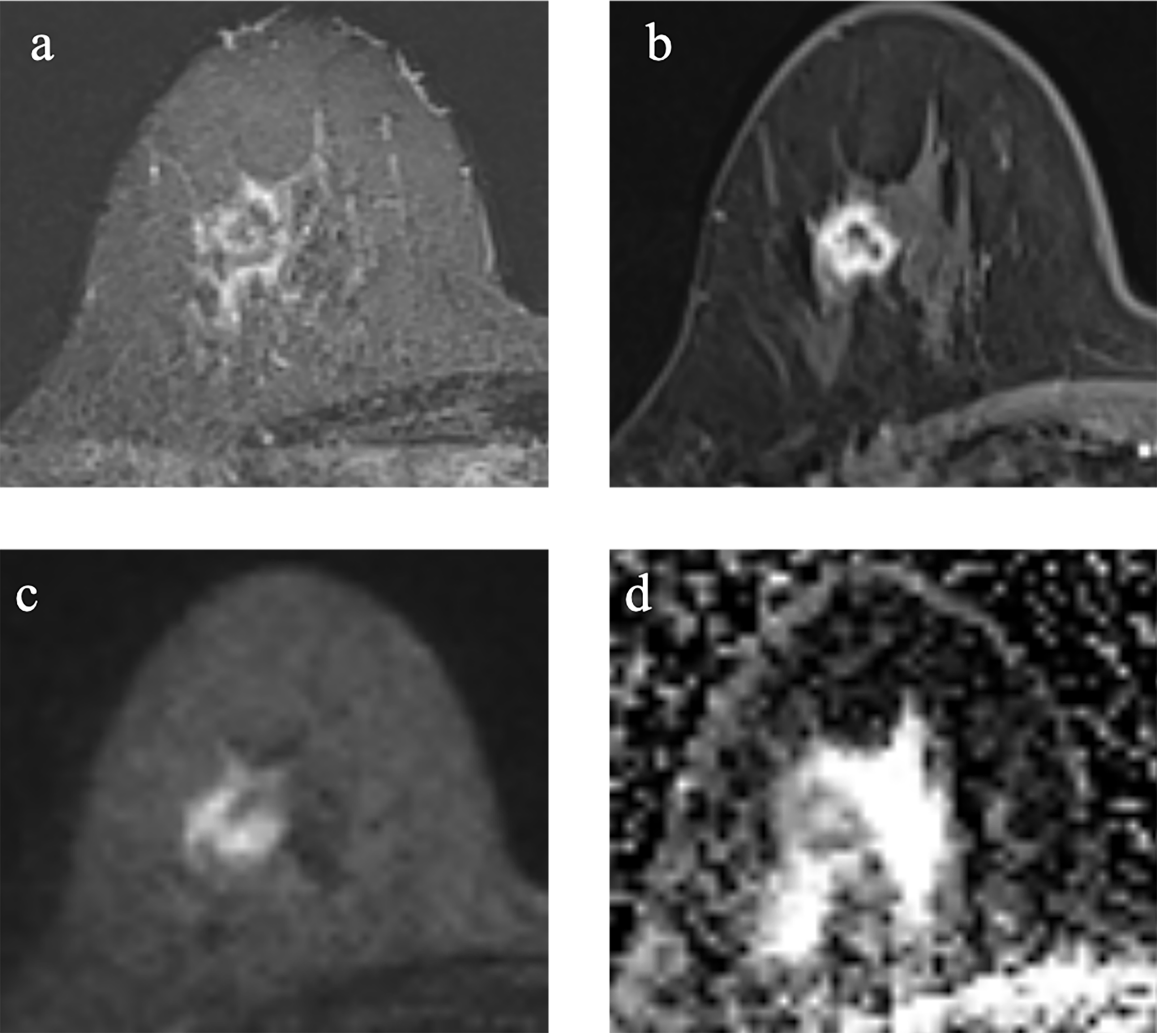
Example images of a 70-year-old woman with TNBC without TP53 mutation in the right breast. (A-D). (A): T2-weighted-image. (B): the first phase of T1WI after contrast enhancement. (C): diffusion-weighted image of b_1000_. (D): apparent diffusion coefficient (ADC) map.
